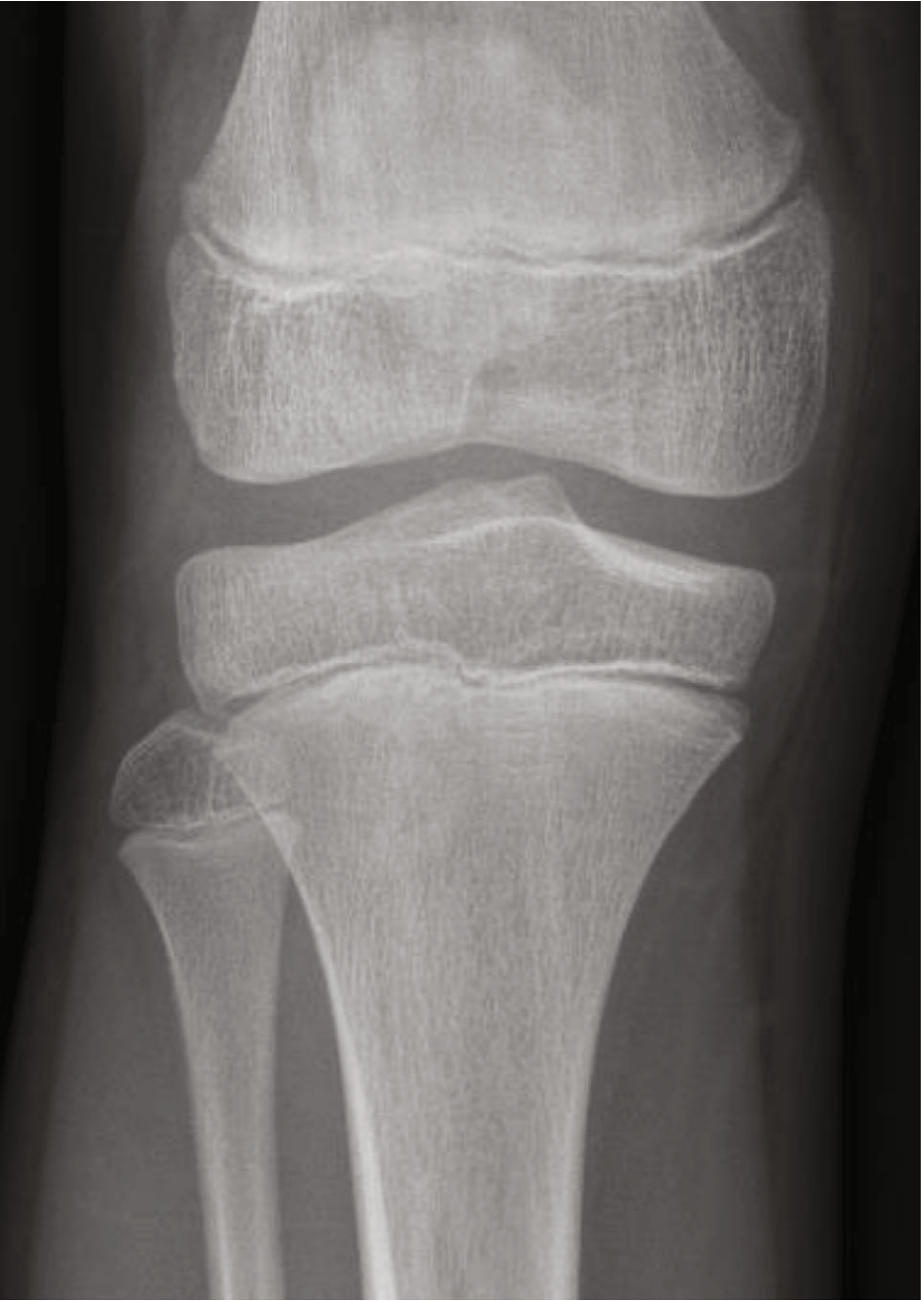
X-ray 2. AP view of the right knee, initial radiological findings.
